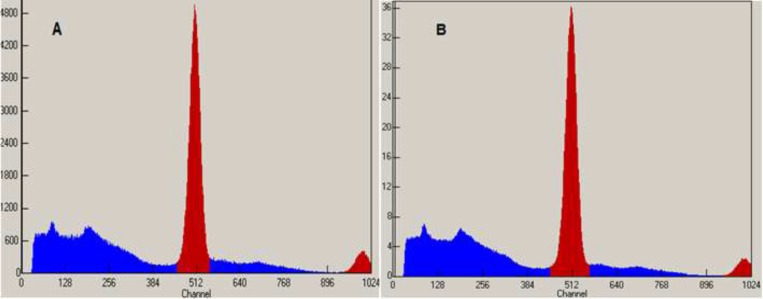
TLC Scanner Chromatograms of ^18^F-FDG after synthesis (A) and 10 h later (B) at r.t.
